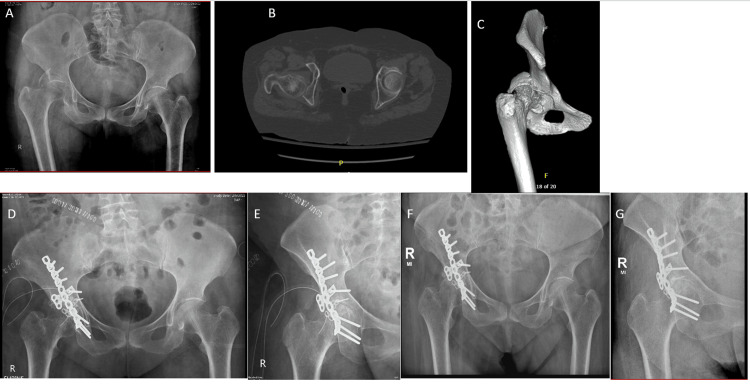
Radiological progression of a 60-year-old male with good outcome: initial presentation, post-operative (6 weeks), and 6-month follow-up images A 60-year-old male presented following a car-on-car road traffic accident, resulting in a right acetabulum fracture with involvement of the anterior column. Fig. A: Initial X-ray revealing a right acetabulum fracture involving the anterior column. Fig. B: Obturator view X-ray of the right acetabulum confirming the anterior column fracture. Fig. C: Axial CT scan of the hip showing a clear anterior column fracture. Fig. D: Sagittal CT scan demonstrating the same anterior column fracture. Fig. E: 3D reconstruction from a CT scan providing detailed visualization of the anterior column fracture. Fig. F: Postoperative X-ray showing the fixation of the fracture. Fig. G: X-ray taken 6 months post-fixation, assessing the healing and alignment of the fracture
